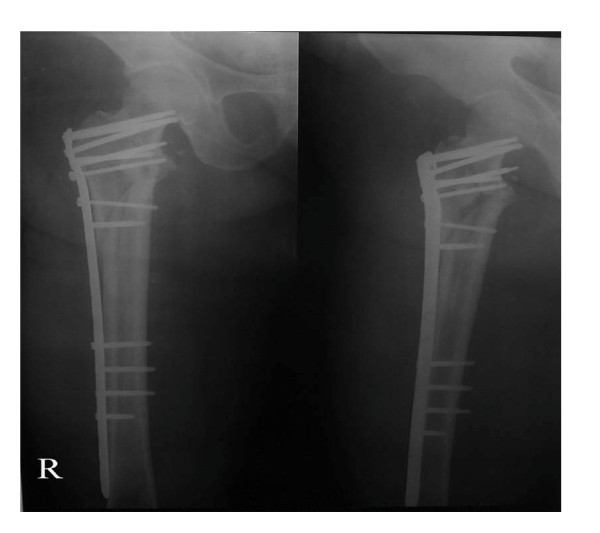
Postoperative AP and lateral Radiographs of the unstable intertrochanteric fracture stabilized by reverse LISS plating.
